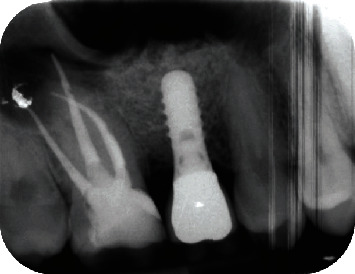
Periapical radiograph obtained one year after implant placement.
